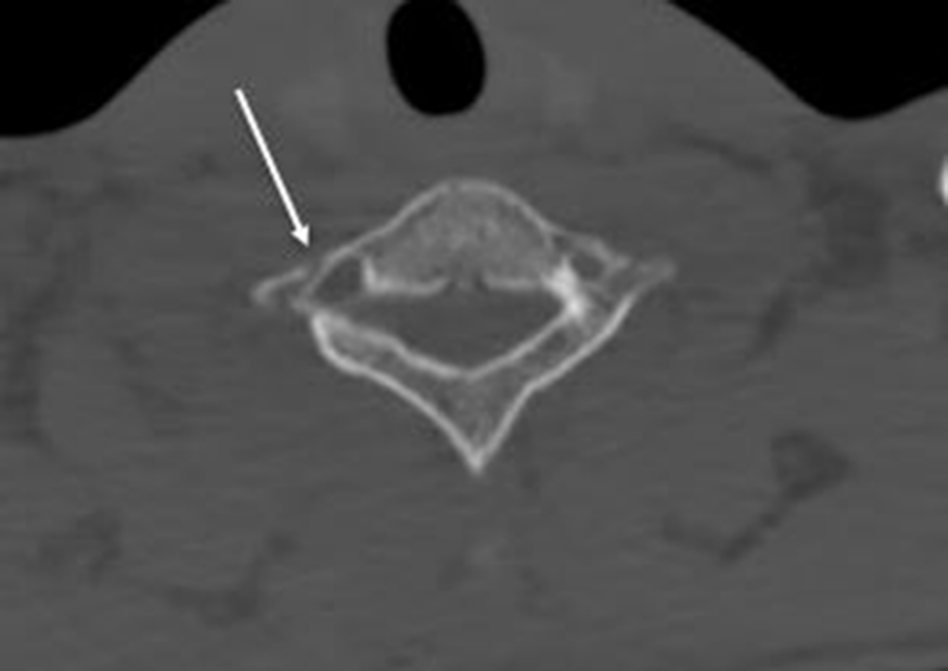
CT axial cut shows right fracture transverse process of C7.
